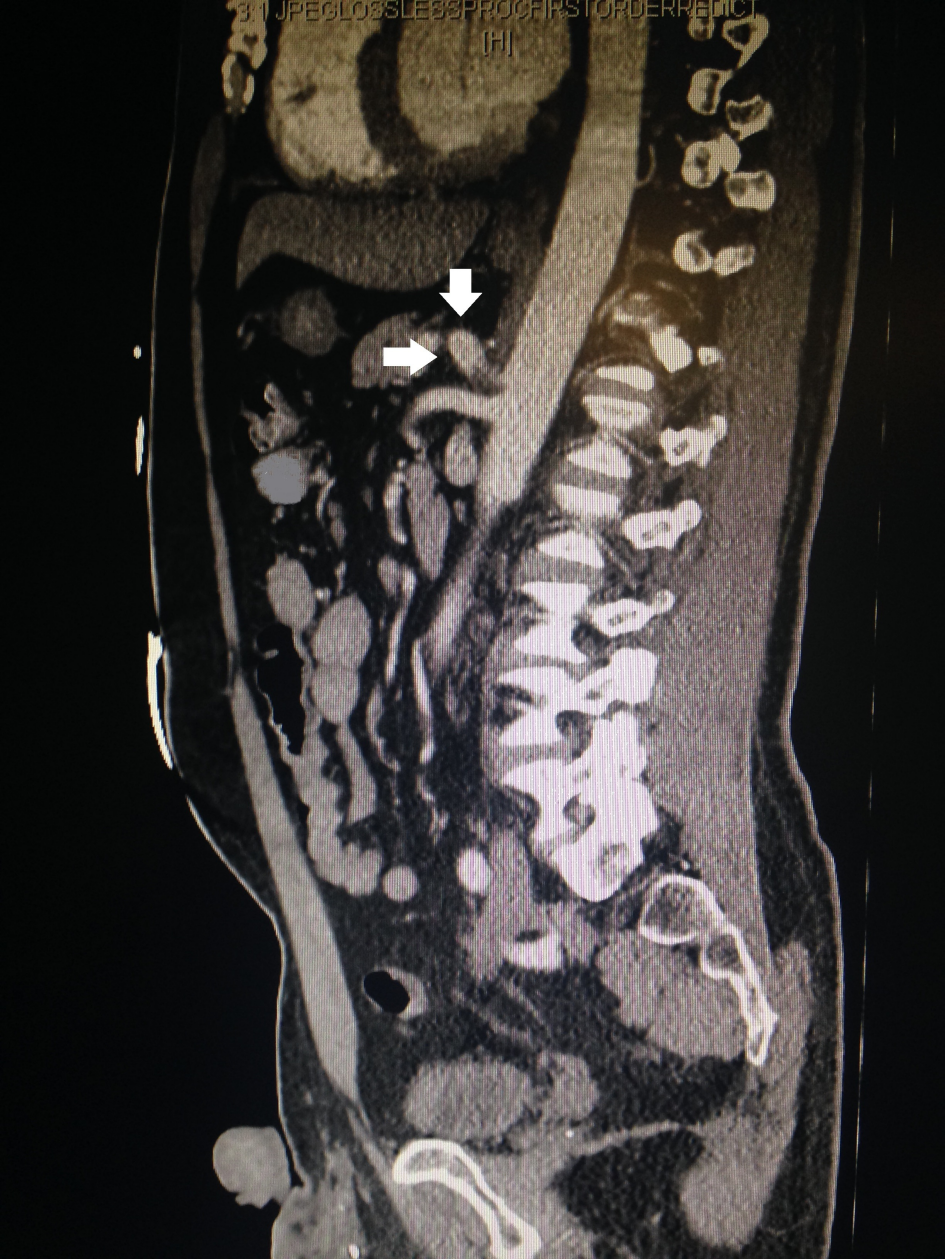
Computed tomography (CT) of abdomen and pelvis with contrast. Pseudoaneurysm involving the ostium of the celiac artery (arrows) with focal dissection.
